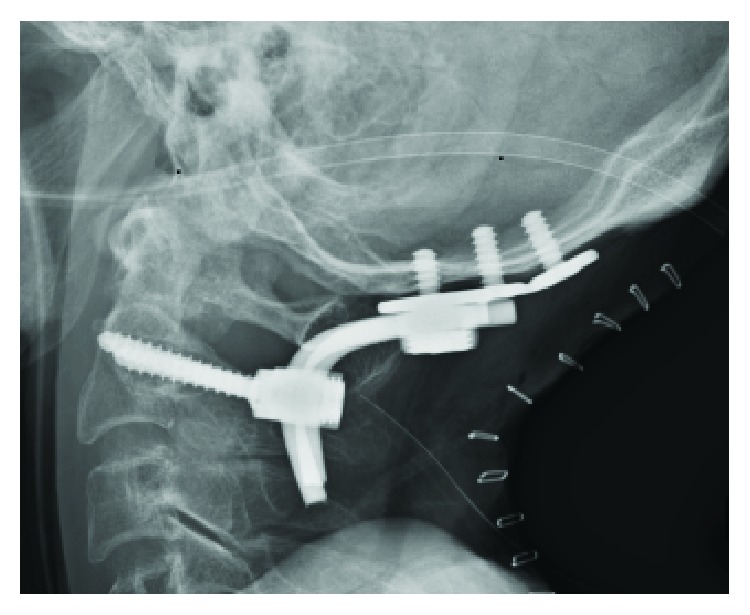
Postoperative radiograph shows occipitocervical fusion achieved by an occipital plate and bilateral C2 pedicle screws.
